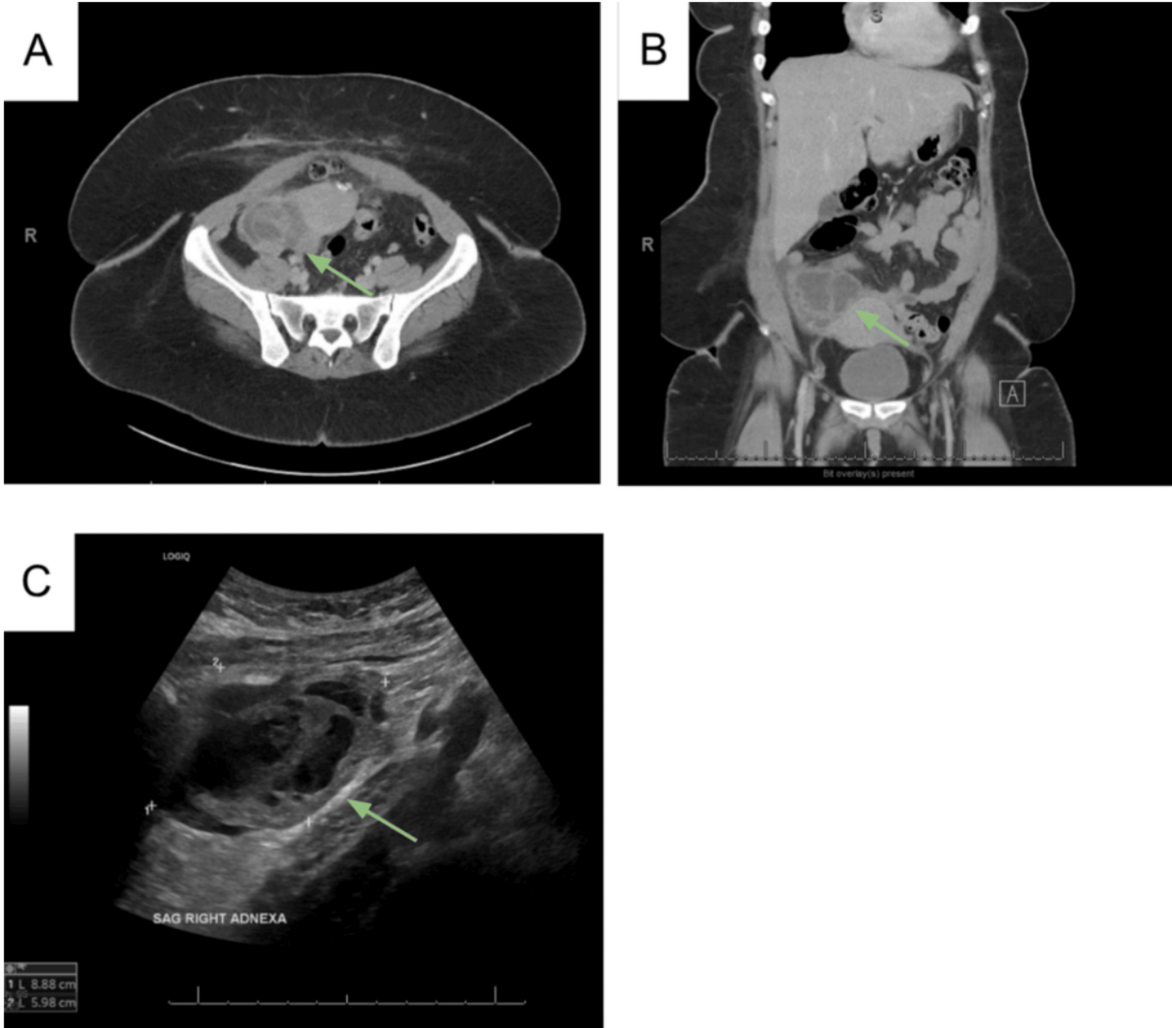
CT and ultrasound on initial presentation. CTAP with contrast showed a multiloculated right adnexal mass measuring 7.5 x 2.5 x 5.8 cm, concerning for a TOA and small calcified fibroid [axial (A) and coronal (B) planes]. Small right lower abdominal and pelvic free fluid. Pelvic ultrasound (C) showing a complex heterogeneous solid and cystic structure in the right adnexa measuring up to 8.9 x 6.0 x 8.1 cm with internal vascularity. CTAP, CT of the abdomen and pelvis; TOA, tubo-ovarian abscess
